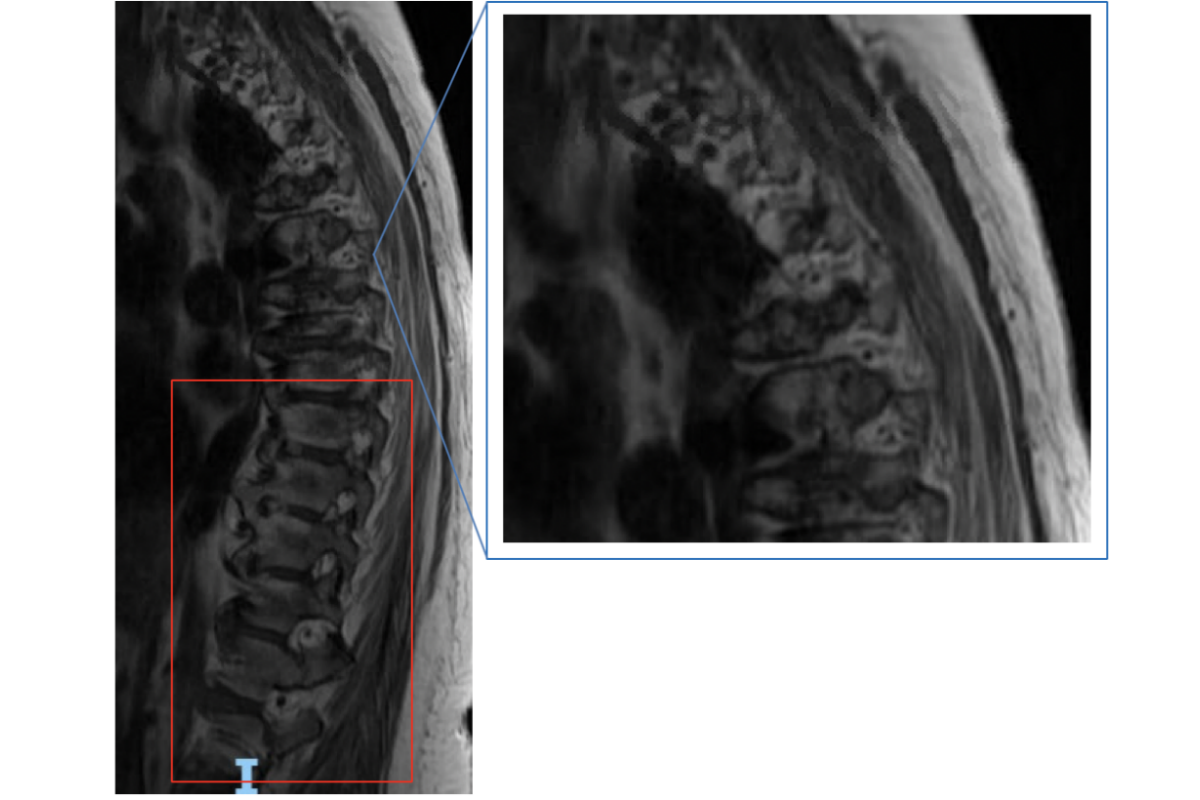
T1-weighted sagittal magnetic resonance imaging of the cervical and thoracic spine revealing C4-C5 osteophyte complex with associated C2-C7 spinal cord compression of the thecal sac secondary to disc osteophyte complexes (blue box) and disseminated idiopathic skeletal hyperostosis syndrome throughout the thoracic spine, particularly at the levels of T5-T9 (red box). Orientation: I indicates inferior.
